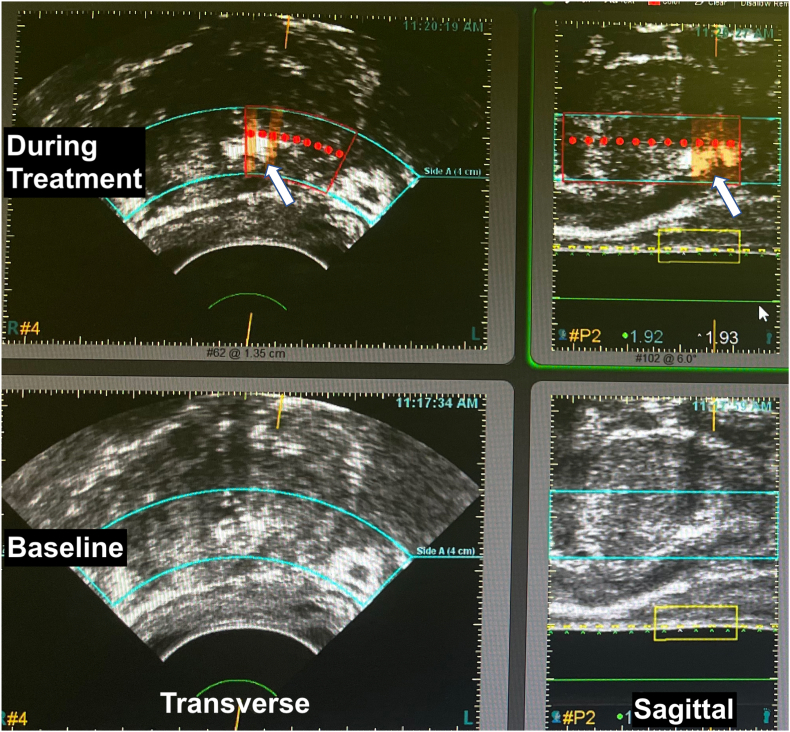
Images from screen of HIFU device (Sonablate 500) during focal therapy (partial gland ablation of left-sided tumor). Lower panel shows prostate before treatment started; upper panel shows treatment in progress; left side, transverse images; right side sagittal. PSMA hotspot (see Fig. 1) helped provide cognitive co-registration. Red dots indicate HIFU treatment foci at mid-zone of prostate. Note ‘popcorn’ effect (arrows), indicating cavitation created by treatment. (For interpretation of the references to colour in this figure legend, the reader is referred to the Web version of this article.)
